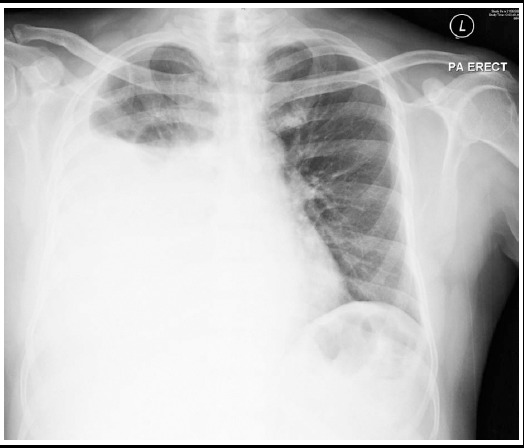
Chest X-ray shows significant right pleural effusion.
